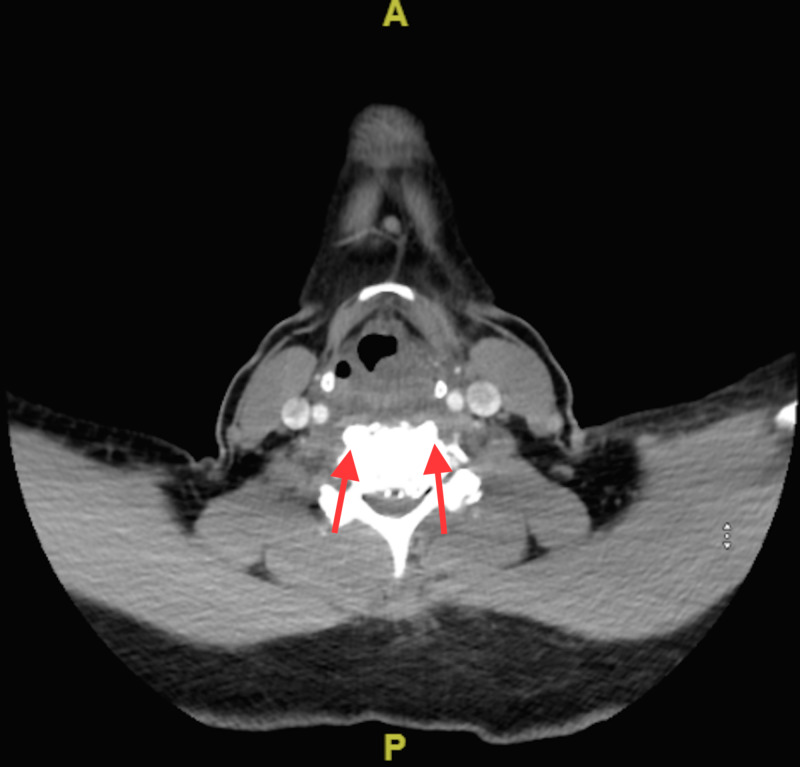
Axial view of CT cervical spine showing calcifications of the longus colli (arrows), appreciated on the anterior aspect of the vertebrae
